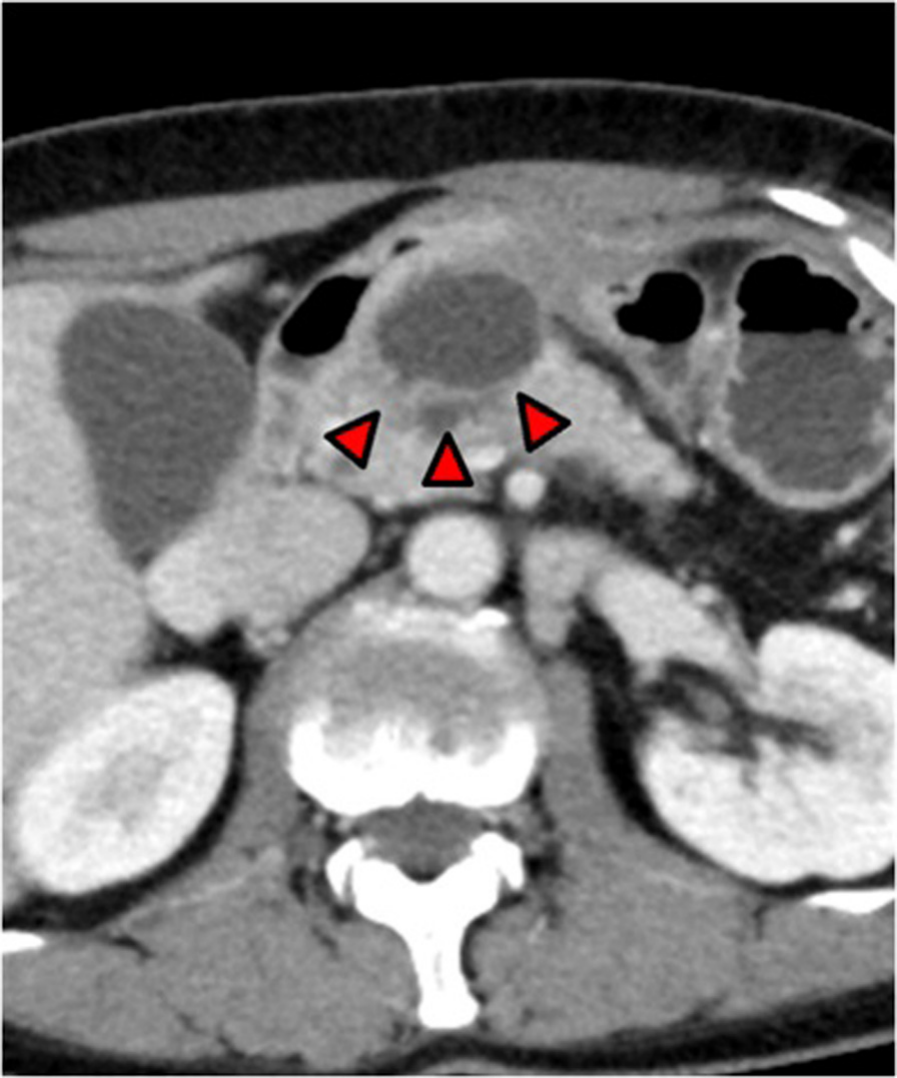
Enhanced CT scan. The cyst exhibited extrapancreatic growth with dilatation of the main pancreatic duct. The low-density area indicated by the arrows is the main pancreatic duct. There was neither a mass lesion nor a low-density area suggestive of pancreatic cancer.
